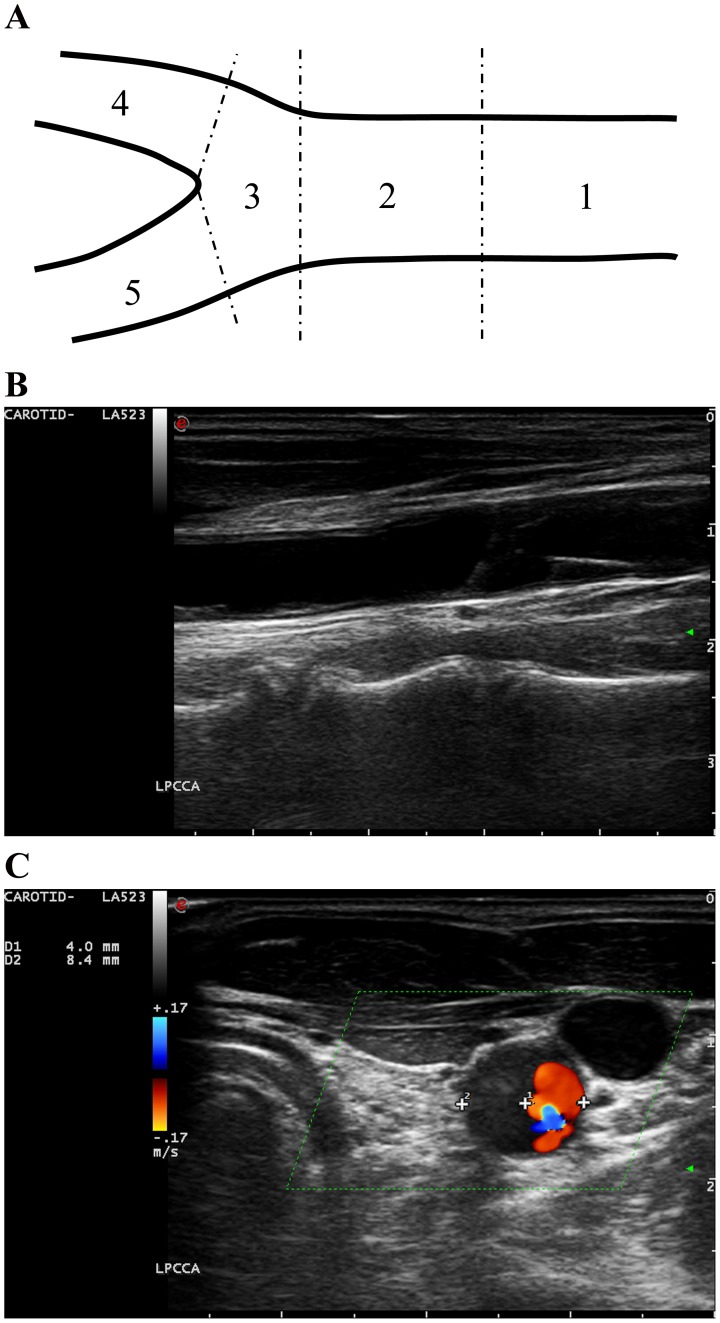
Assessment of carotid plaque burden. A). Five segments of the extra-cranial carotid artery: 1. proximal common carotid artery (≥2 cm proximal to carotid bifurcation); 2. distal common carotid artery (<2 cm proximal to carotid bifurcation); 3. carotid bulb and bifurcation; 4. internal carotid artery; and 5. external carotid artery. B). A longitudinal gray-scale image of a carotid plaque in the proximal common carotid artery. C). A transverse duplex ultrasound (gray-scale+Doppler) image of the carotid plaque in B. The plaque is echolucent with a unclear boundary between the lumen and the plaque. Therefore, Doppler ultrasound is applied to demonstrate the lumen at the stenosis. The degree of the carotid stenosis is expressed as the percentage reduction of lumen diameter (1-D1/D2 = 52.4%). Carotid plaque score is the summation of the degree of carotid stenosis of the five segments in both carotid arteries.
